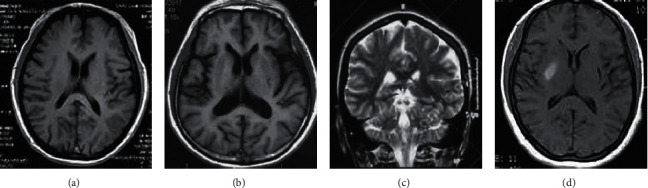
MRI image of patients with ACI. (a) MRI image of normal human brain; (b) MRI image of posterior cortical cerebral infarction; (c) MRI image of cerebellar watershed infarction; (d) MRI image of intracerebral cerebral infarction.
